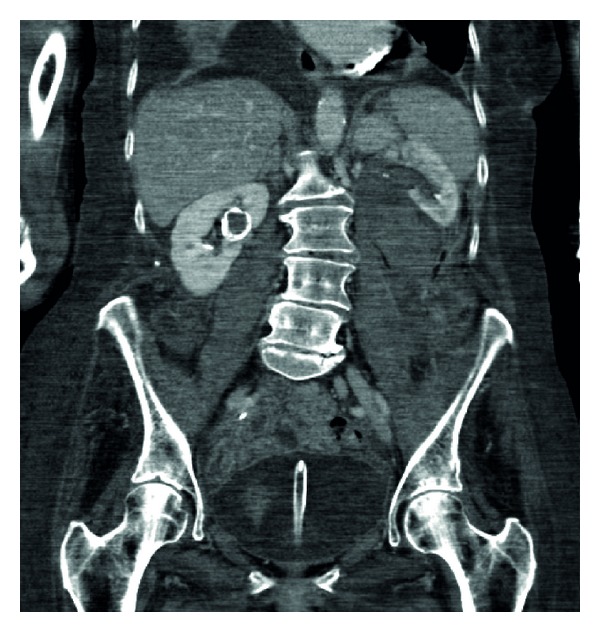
Split bolus contrast CT KUB: coronal section, left kidney with hydronephrosis and right kidney calcification.
